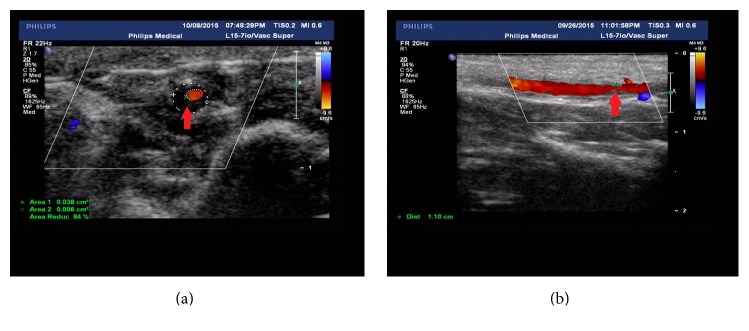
Color Doppler ultrasound demonstrating the atherosclerotic plaque in the ICA. The red arrows in (a) and (b) point to the places where the internal carotid atherosclerotic plaques are located.
